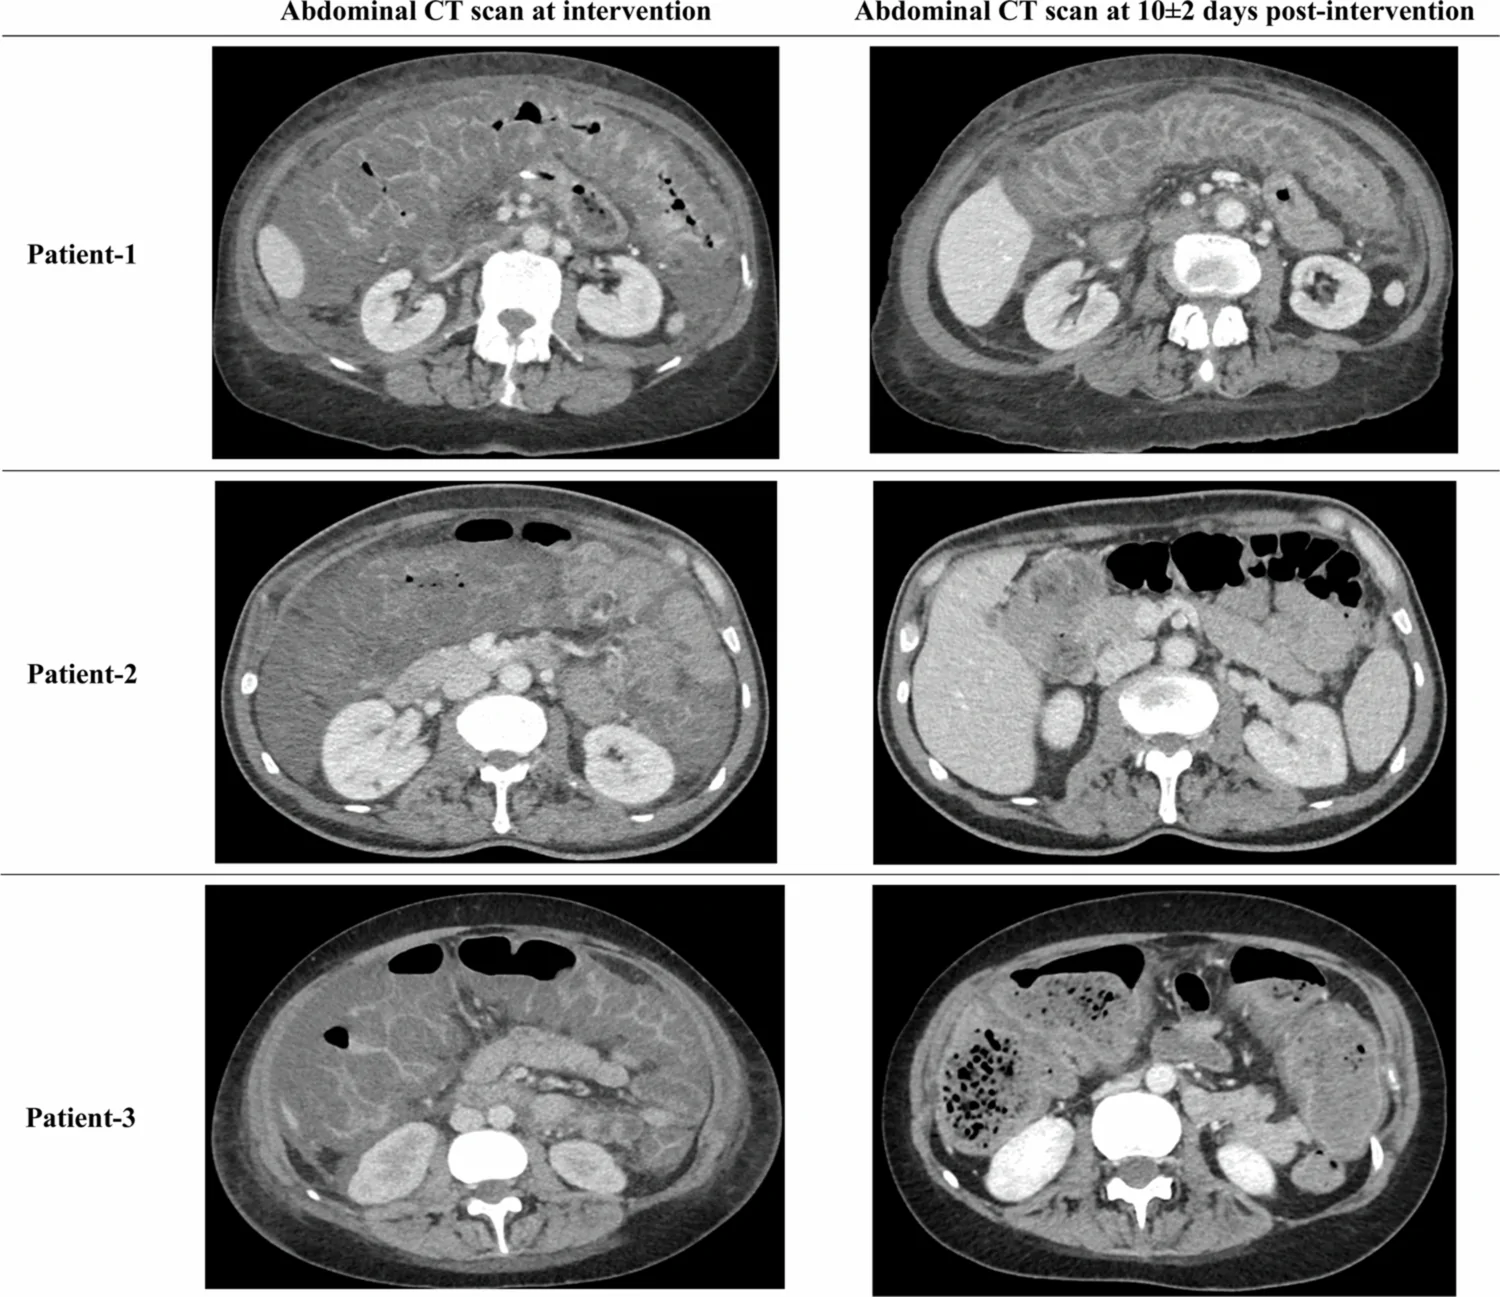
Representative contrast-enhanced abdominal CT images acquired in the horizontal plane at the level of the transverse colon in study participants.
